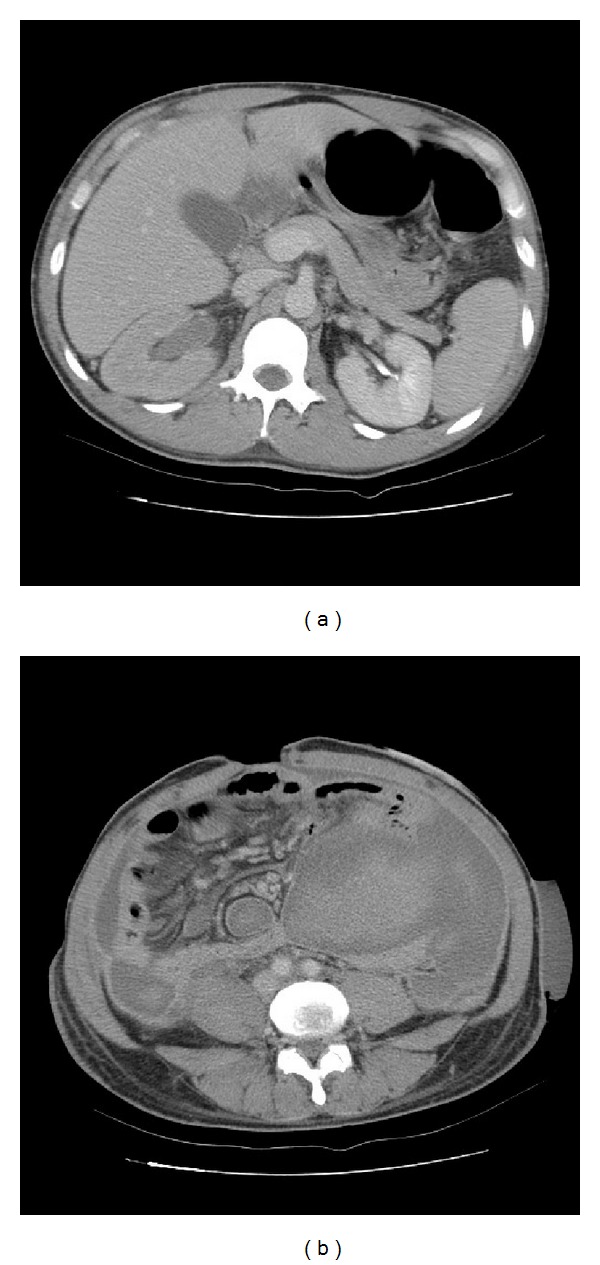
Computerized tomography (CT) of abdomen revealed (a) mild right hydronephrosis and hydroureter at initial presentation and (b) a big hypovascular tumor in lower abdomen area, with bowel loop right shifting. Multiple enlarged LNs were also noted at para-aortic region.
